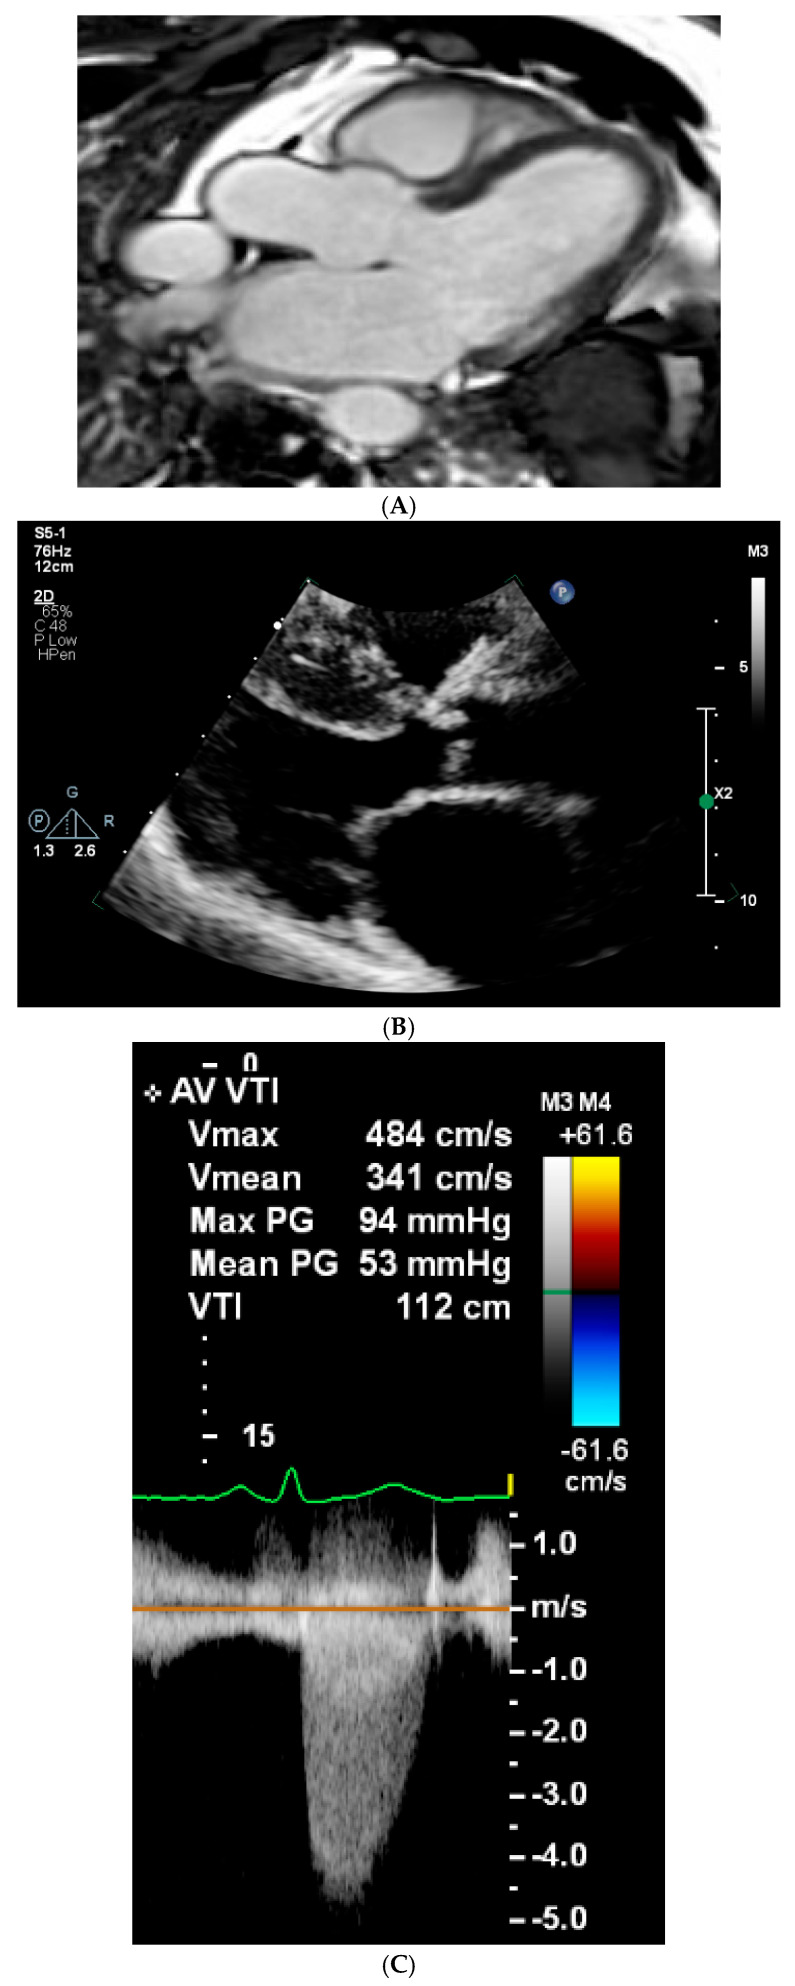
Cardiac manifestations in patients with attenuated MPS II (A) and MPS VI (B,C). (A) Cardiac magnetic resonance imaging (MRI) in a patient with attenuated MPS II demonstrates focal myocardial fibrosis in the basal to mid-lateral wall of the left ventricle. Fibrotic changes are consistent with glycosaminoglycan (GAG) accumulation and support subclinical myocardial involvement despite minimal valvular abnormalities. (B) A transthoracic echocardiogram (parasternal long axis (PLAX) view) in a patient with MPS VI demonstrating GAG-related infiltration of the aortic valve and severe aortic stenosis. This PLAX view shows marked thickening and restricted mobility of the aortic valve leaflets, consistent with severe aortic stenosis. The echocardiographic findings reflect GAG accumulation within valvular tissue, a recognised cardiac manifestation in MPS VI. (C) A transthoracic echocardiogram (continuous-wave Doppler) in a patient with MPS VI demonstrating haemodynamically severe aortic stenosis. Continuous-wave Doppler tracing across the aortic valve shows a peak velocity (Vmax) of 4.84 m/s and a mean pressure gradient of 53 mmHg, with a peak gradient of 94 mmHg. The velocity time integral (VTI) is 112 cm. These values are consistent with severe aortic stenosis and reflect the functional consequence of GAG infiltration of the valve apparatus.
